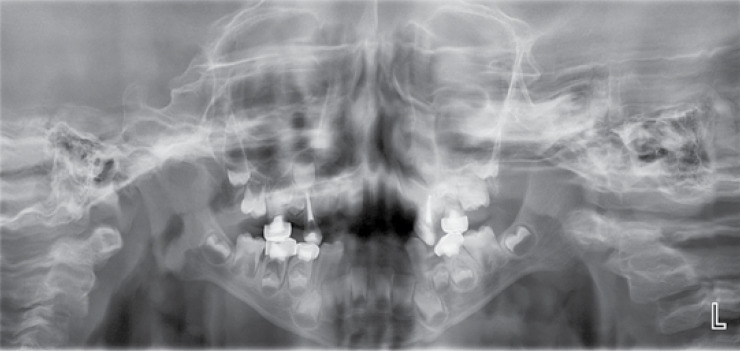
A panoramic radiographic image indicating the treated deciduous teeth and hypoplasia of permanent teeth
